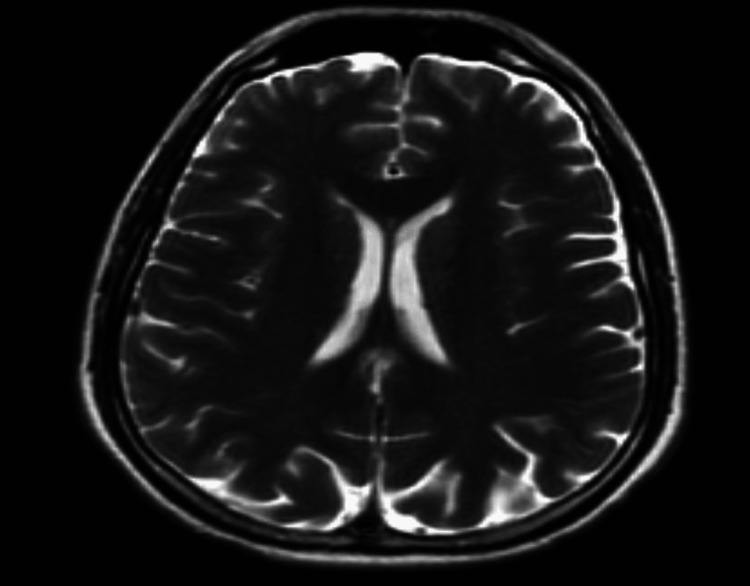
Initial brain MRI of a patient with West Nile encephalitis showing no abnormalities.
